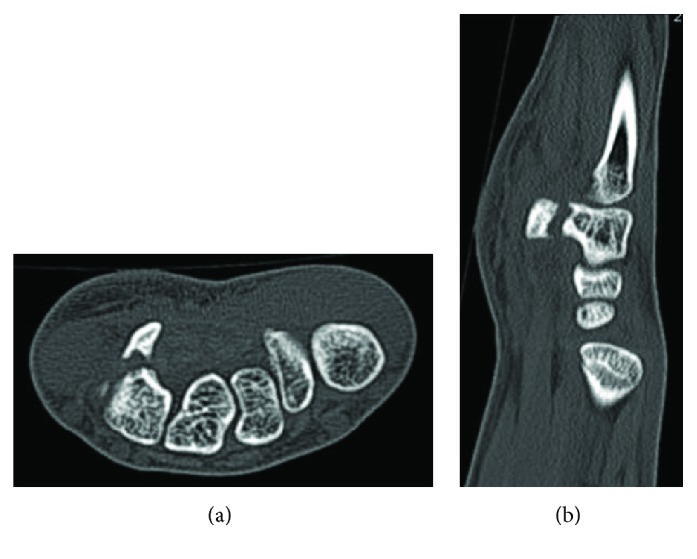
Computed tomography (CT) images. (a) Axial CT image. (b) Sagittal CT image.
